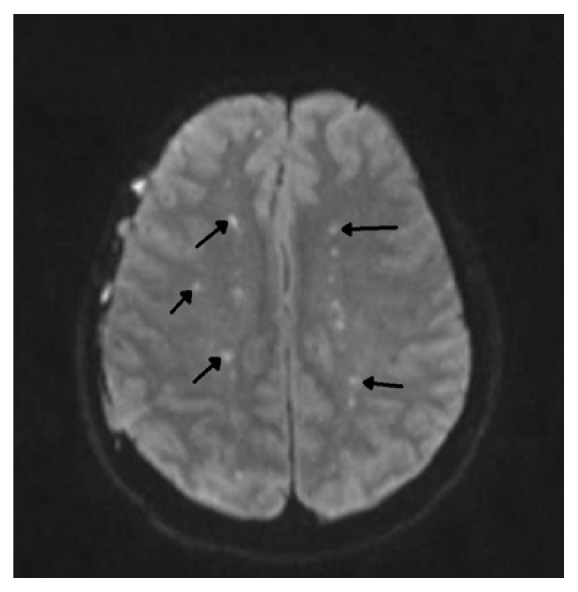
Brain MRI revealed multiple bilateral acute cerebral infarcts (black arrows) with restricted diffusion areas in a “starfield” pattern favoring fat emboli.
